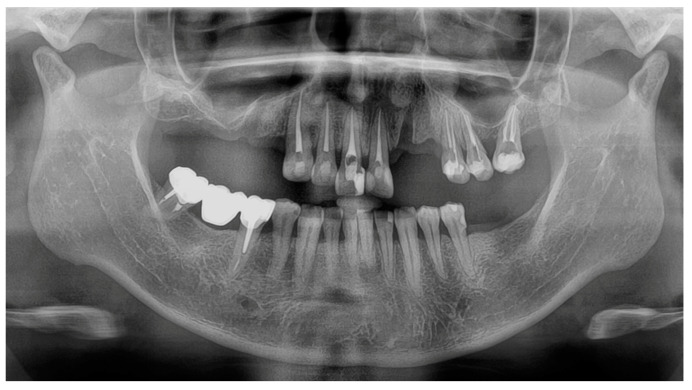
Initial panoramic radiography.
